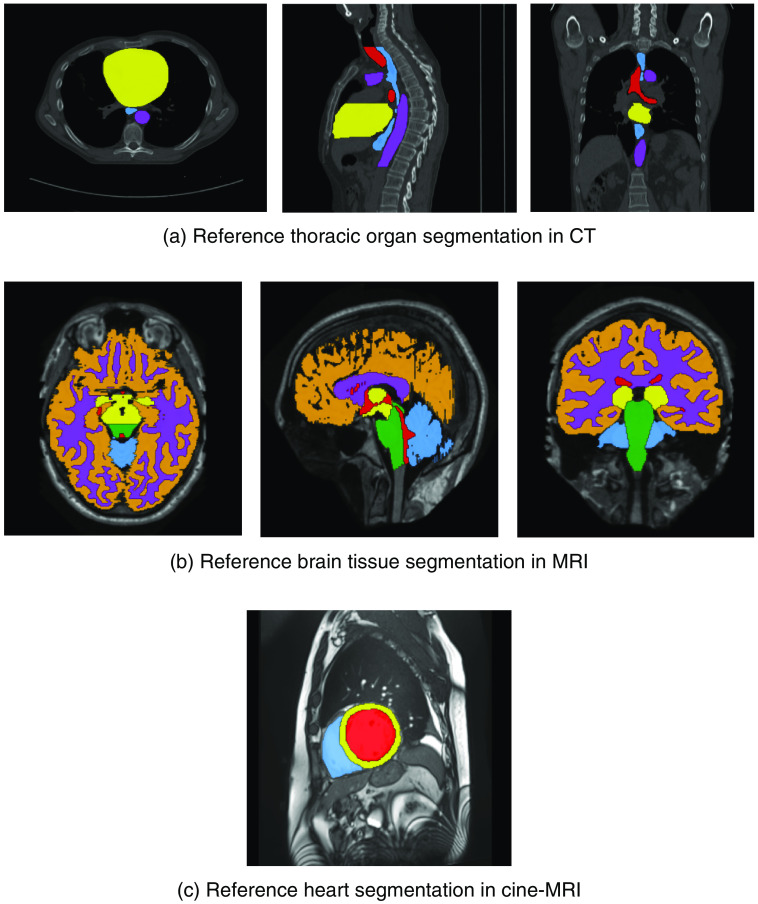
Example of the three datasets that were used to train and evaluate ensembles and knowledge distillation. (a) An axial, sagittal, and coronal slice from a CT scan, provided by the ISBI 2019 challenge on SegTHOR in CT images, in which the corresponding reference segmentation of the aorta (purple), trachea (red), heart (yellow), and esophagus (blue) are shown. (b) An axial, sagittal, and coronal slice from an MRI, provided by the MICCAI 2012 challenge on multi-atlas labeling in brain MRI, in which the corresponding reference segmentation of the cGM (orange), BS (green), WM (purple), vCSF (red), BGT (yellow), and CB (blue) are shown. (c) Short-axis view of the heart from an end-diastole cine-MRI, provided by the MICCAI 2017 automated cardiac diagnosis challenge, in which the corresponding reference segmentation of the left ventricle (red), myocardium (yellow), and right ventricle (blue) are shown.
